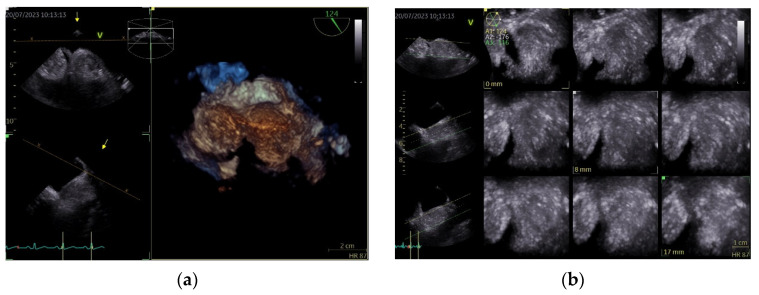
Transesophageal echocardiography, tridimensional reconstruction. (a) Four-dimensional zoom acquisition revealing two so-called masses attached to the IAS with the appearance of distinct structures, bulging in the RA (inferior) and imprinting the LA surface of the IAS (superior); (b) 4D zoom acquisition after multislice analysis, confirming the homogenous aspect of the “structures”.
